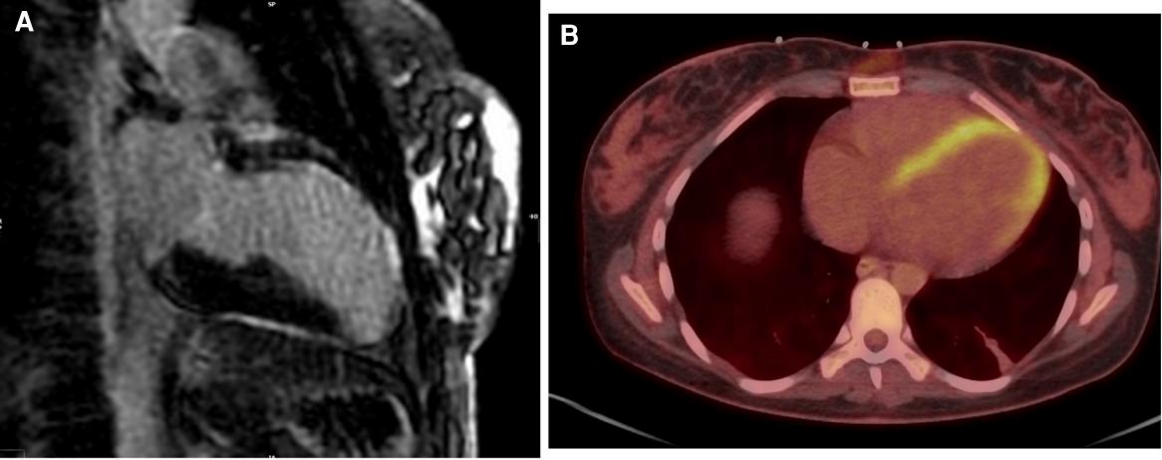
Tissue viability studies. (*A*) Cardiac magnetic resonance imaging scan showing extensive, late gadolinium enhancement primarily affecting the anterior, anteroseptal, and apical segments. (*B*) Positron emission tomography scan showing global reduction (but not absence) of glucose metabolism in the left ventricle.
